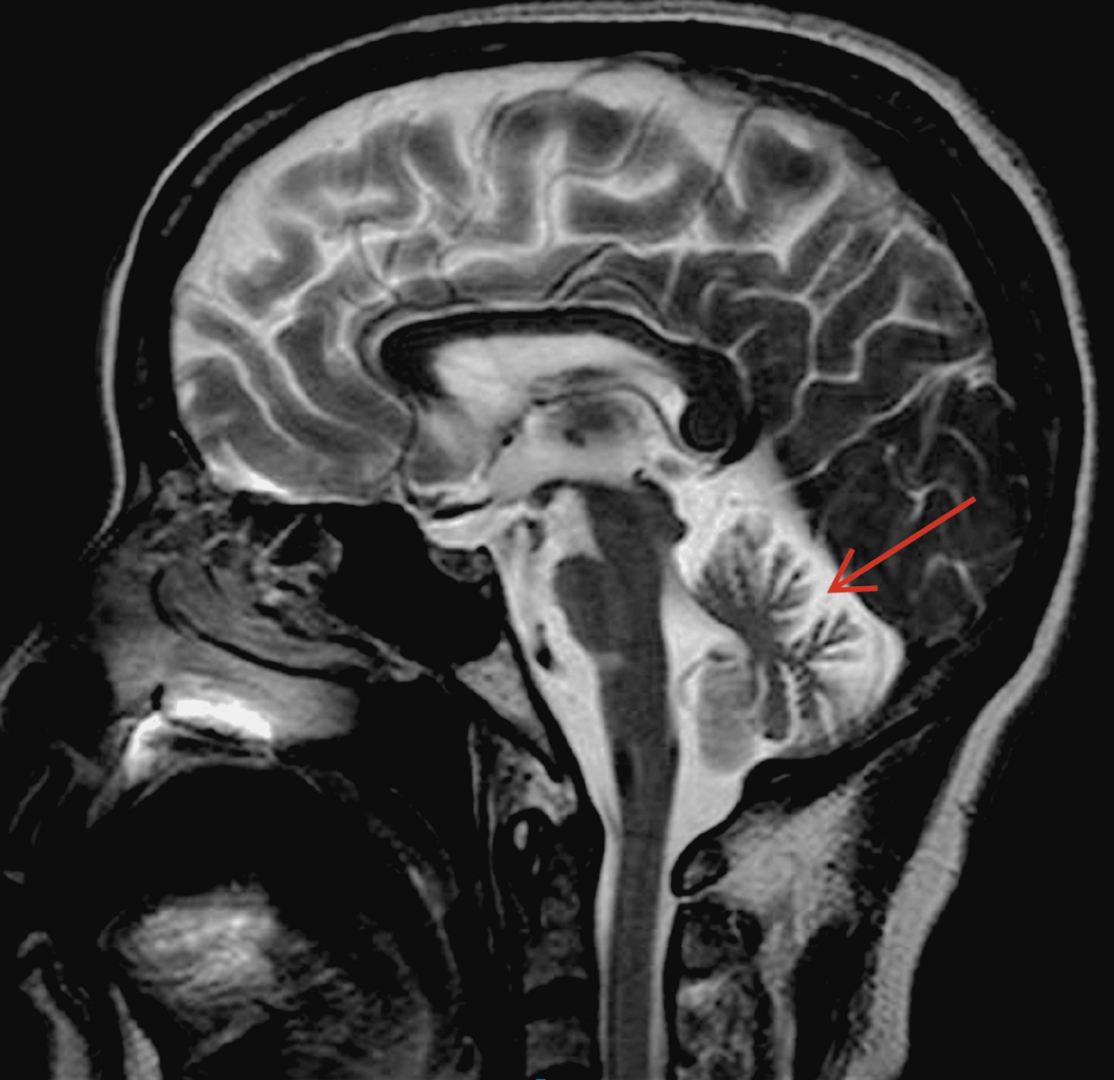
Marked cerebellar atrophy on cranial MRI (sagittal view)
